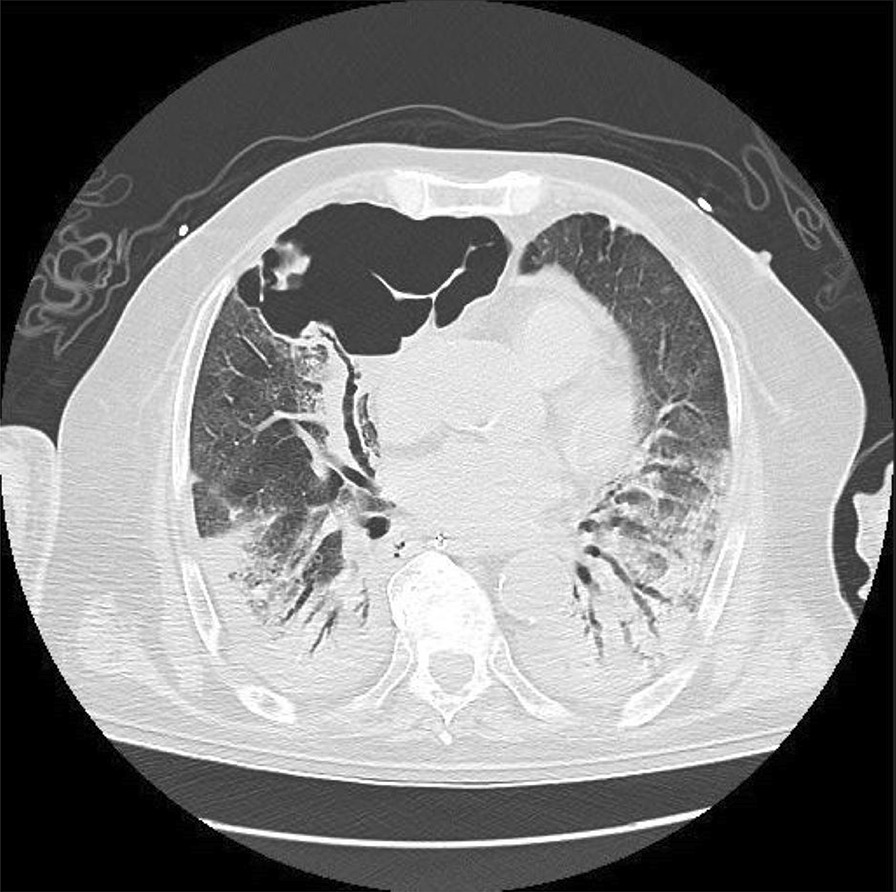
Fig. 1 (abstract P079). CT finding of first case (78 year male). Lung cavity formation during COVID-19 pneumonia
